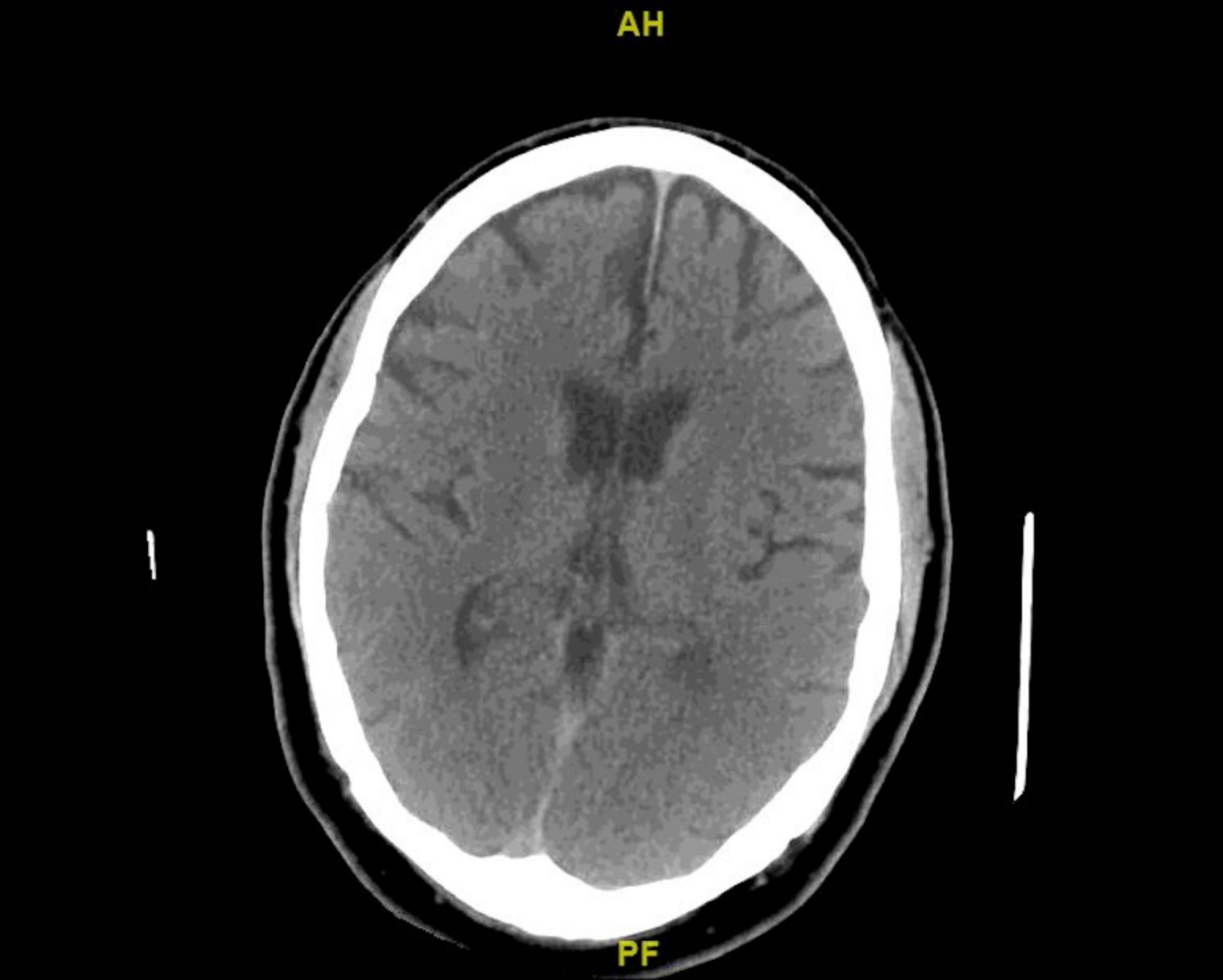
Non-contrast computed tomography (CT) scan of the brain No acute intracranial pathology can be seen in the scan.
